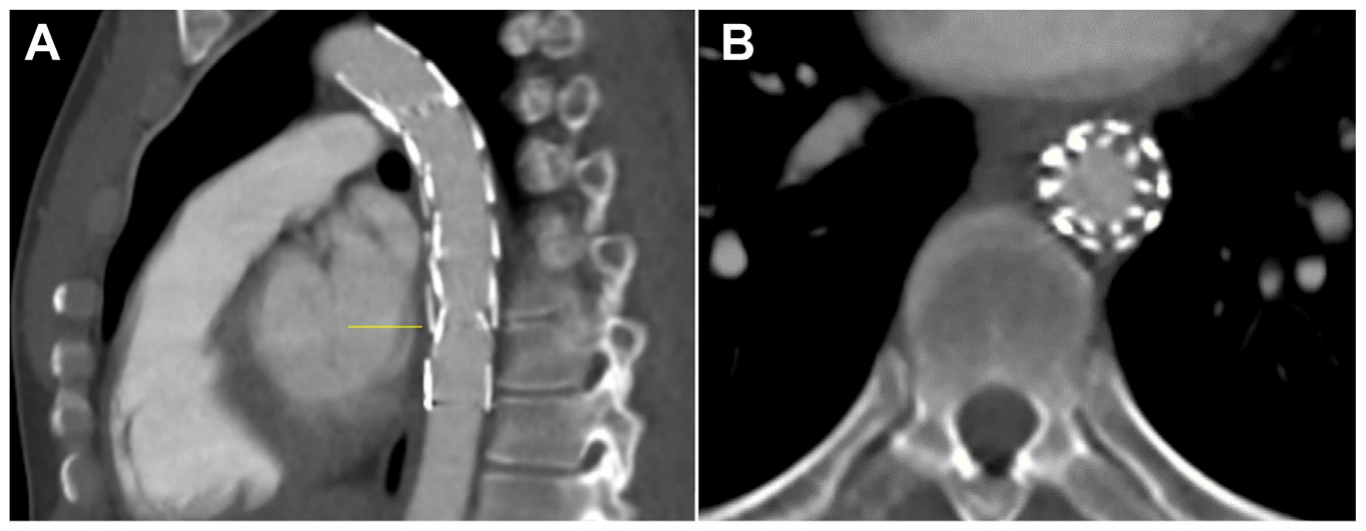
Computed tomography angiography (CTA) after relining of thoracic endovascular aortic repair (TEVAR). A, Sagittal plane. B, Axial plane at distal end of TEVAR (level of *yellow line* in Fig A) showing thrombus compressed behind the overlapping stent graft.
